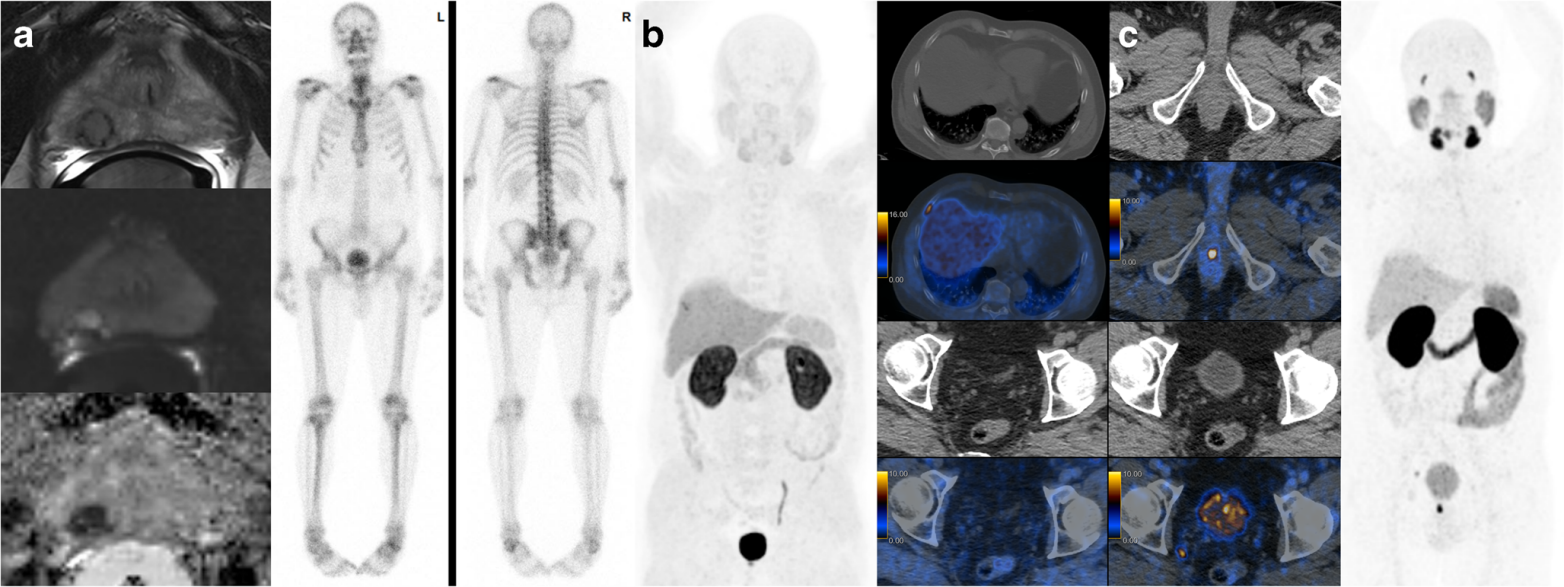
^68^Ga-PSMA-11 positron emission tomography/magnetic resonance imaging (^68^Ga-PSMA-11 PET/MRI) depicts lesions missed by choline PET. (A) Staging prostate MRI (top T2-weighted fast recovery fast spin-echo sequence, middle diffusion-weighted sequence, and bottom apparent diffusion coefficient) and bone scan of a 64-year-old patient with a T1c, Gleason score 4 + 4 = 8 tumor, and initial PSA level of 14.3 ng/ml show a PIRADS 4 lesion without suspicious pelvic lymph nodes nor bone metastasis. The patient underwent radical prostatectomy and had a persistent PSA value of 5.27 ng/ml 7 weeks after the surgery. (B) Subsequent choline PET showed a single suspicious rip lesion that was then treated with radiotherapy causing only a slightly drop of the PSA to 4.2 ng/ml with a subsequent rise to 7.15 ng/ml. (C) Following the PSA rise, ^68^Ga-PSMA-11 PET/CT was performed and showed lymph node metastasis and local recurrence
